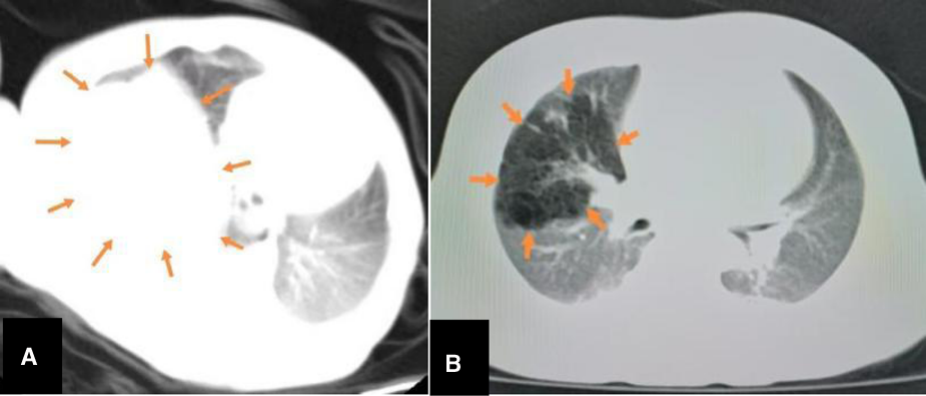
The comparison between CT features FLIT and CPAM. CT shows a mass (arrows) with uniform density in FLIT (A) and a mass (arrows) with low-density cystic spaces in CPAM (B).
